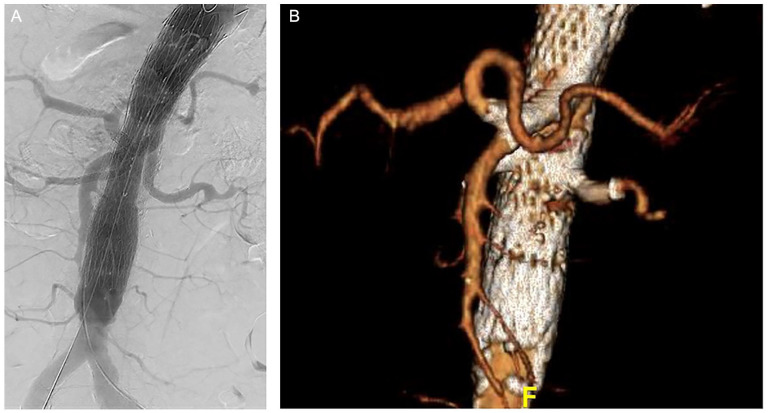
The completion angiography showed patent visceral vessels (Panel A), while the 6-month computed tomography angiography confirmed endograft and bridging stent patency, including the celiac artery fenestration (Panel B).
